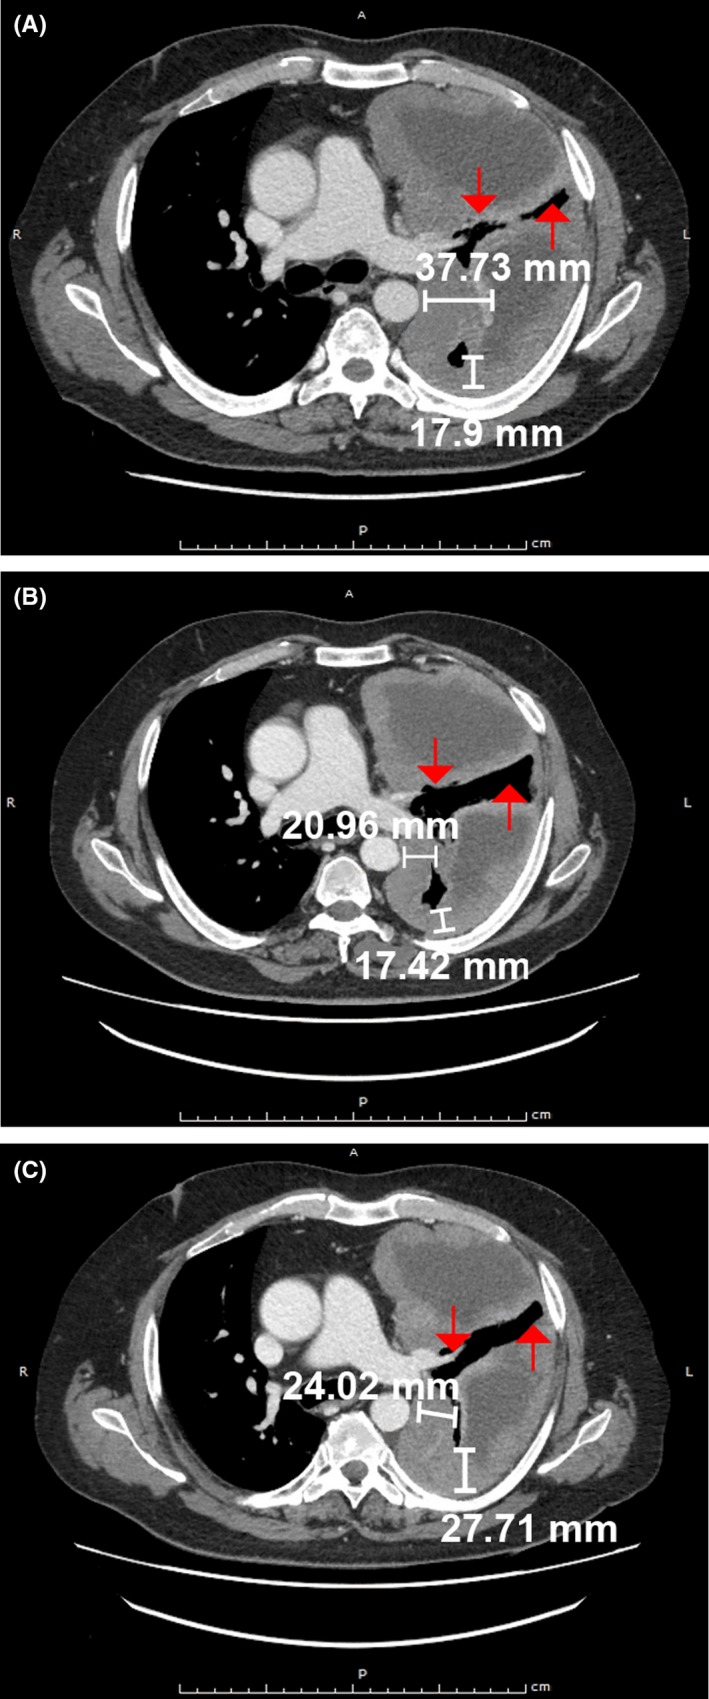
Disease reductions following nintedanib plus pemetrexed rechallenge. A, CT scan prior to nintedanib plus pemetrexed rechallenge. B, CT scan following 59 d of nintedanib plus pemetrexed rechallenge. C, CT scan following 116 d of nintedanib plus pemetrexed rechallenge. Arrows show location of neoplasm. CT, computed tomography; d, days
